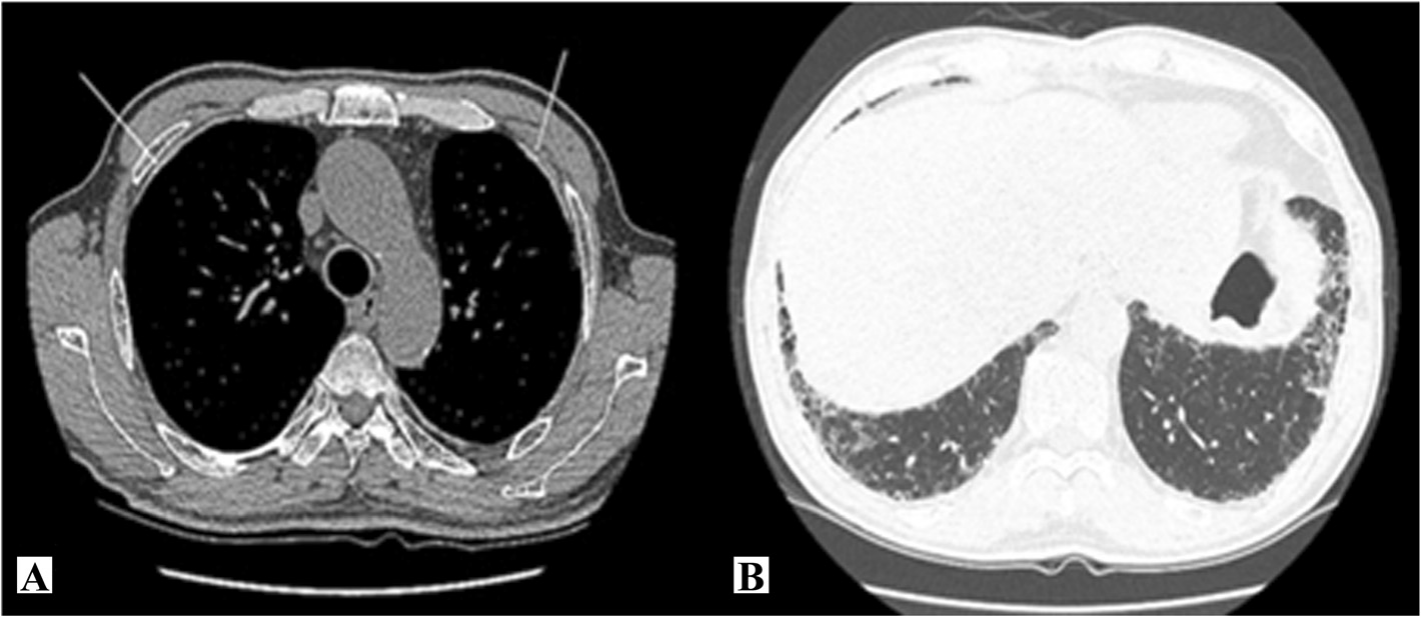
Chest computed tomography images. A. Discrete pleural thickening and calcification in the bilateral hemithorax. B. Fine reticular opacities, ground glass opacity, traction bronchiectasis, and honeycomb appearance in the bilateral lungs.
